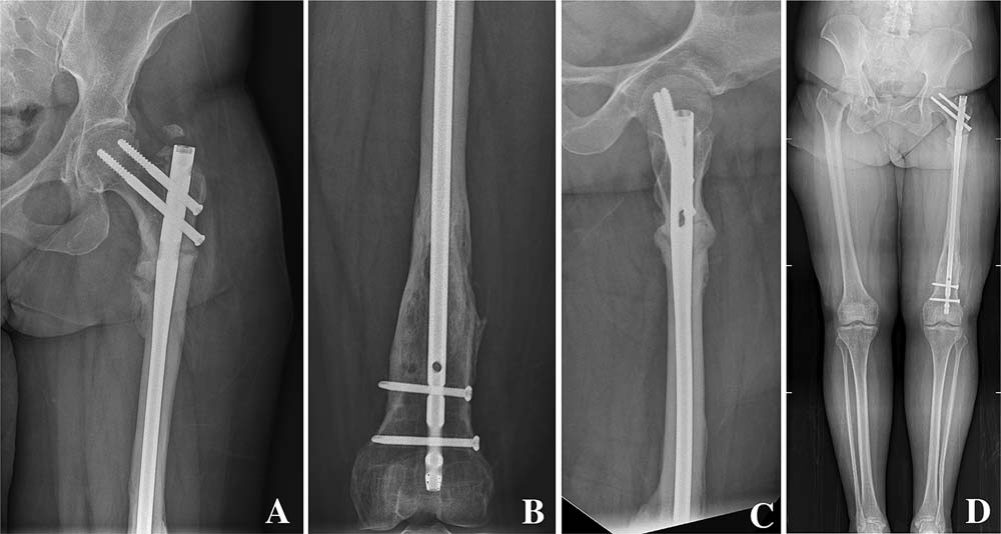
(A, B) Anteroposterior radiographs of the proximal and distal femur showing a correctly positioned antegrade femoral nail inserted via the appropriate proximal entry site. (C) Lateral radiograph of the femur showing stable fixation with appropriate screw placement and no evidence of cortical breach. (D) Scanography of the lower limbs demonstrating restored mechanical alignment.
